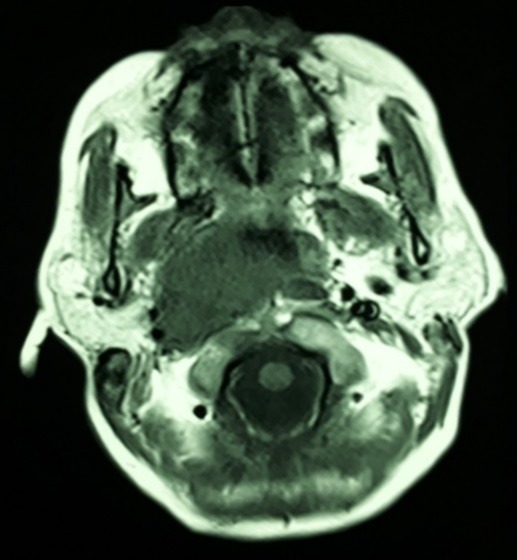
Brain MRI, T2 weighted, axial cut: upward extension of the heterogeneous mass into the middle cranial fossa, that involved the clivus
